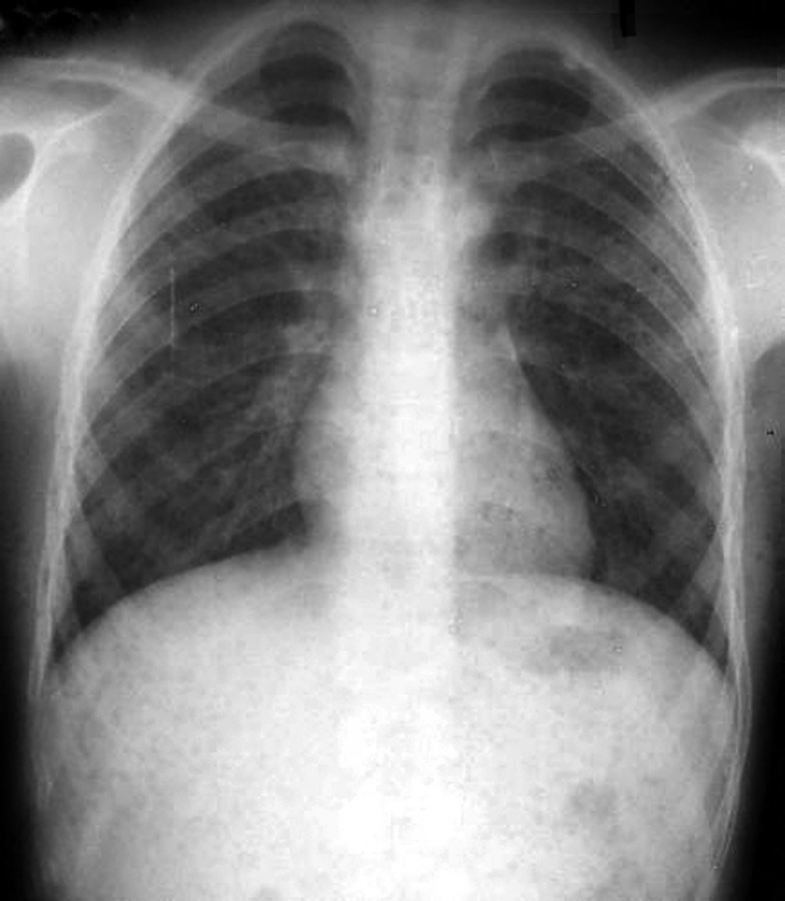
Chest radiograph of patient
